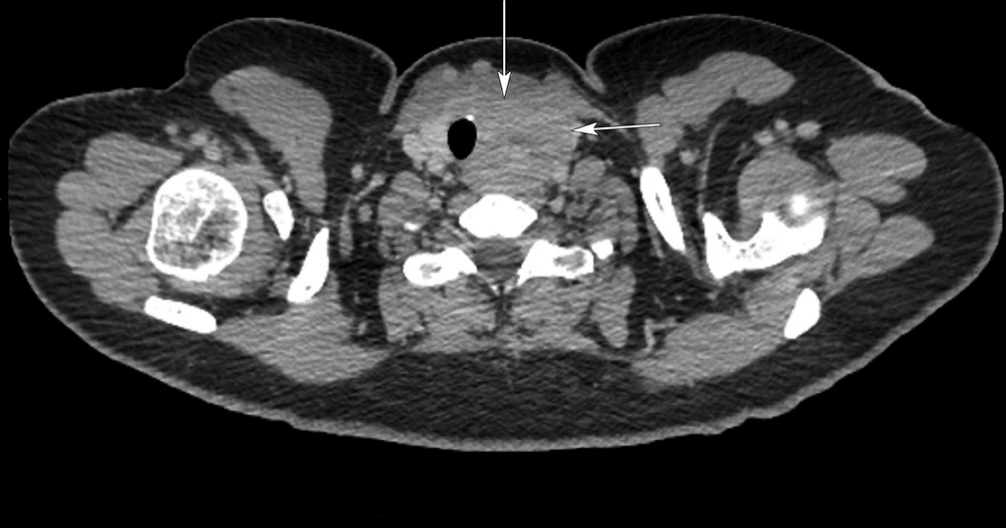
Axial post contrast CT thyroid, shows a poorly defined soft tissue mass in the left thyroid (arrows).
